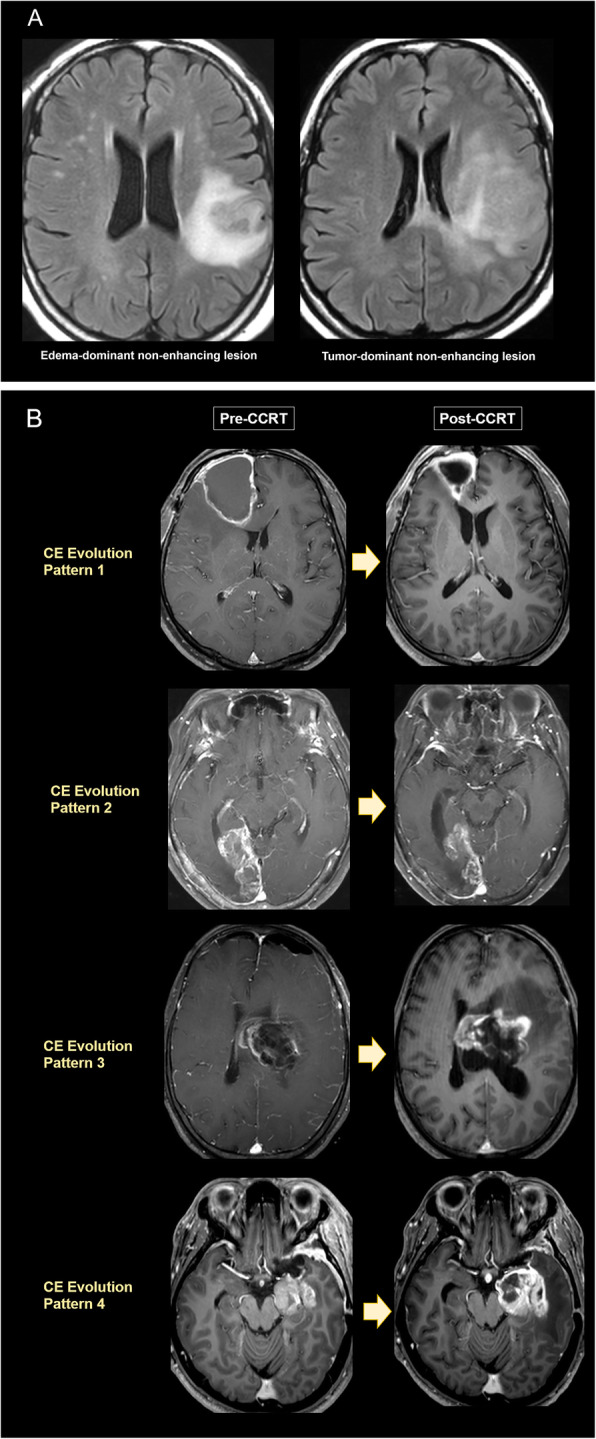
(A) Representative patient images for classification of NEL type based on pre-CCRT T2 FLAIR images. Edema-dominant type NEL (left) and tumor-dominant type NEL (right). (B) Representative patient images for CE evolution pattern based on pre-CCRT (left) and post-CCRT (right) contrast-enhanced T1-weighted MRI. a) new non-measurable CE after GTR (pattern 1); b) stable or responding residual CE after STR (pattern 2); c) new measurable CE after GTR (pattern 3); and d) enlarging residual CE after STR (pattern 4). NEL = non-enhancing lesion; CE = contrast enhancing lesion; CCRT = concurrent chemoradiotherapy; GTR = gross total resection; STR = subtotal resection
